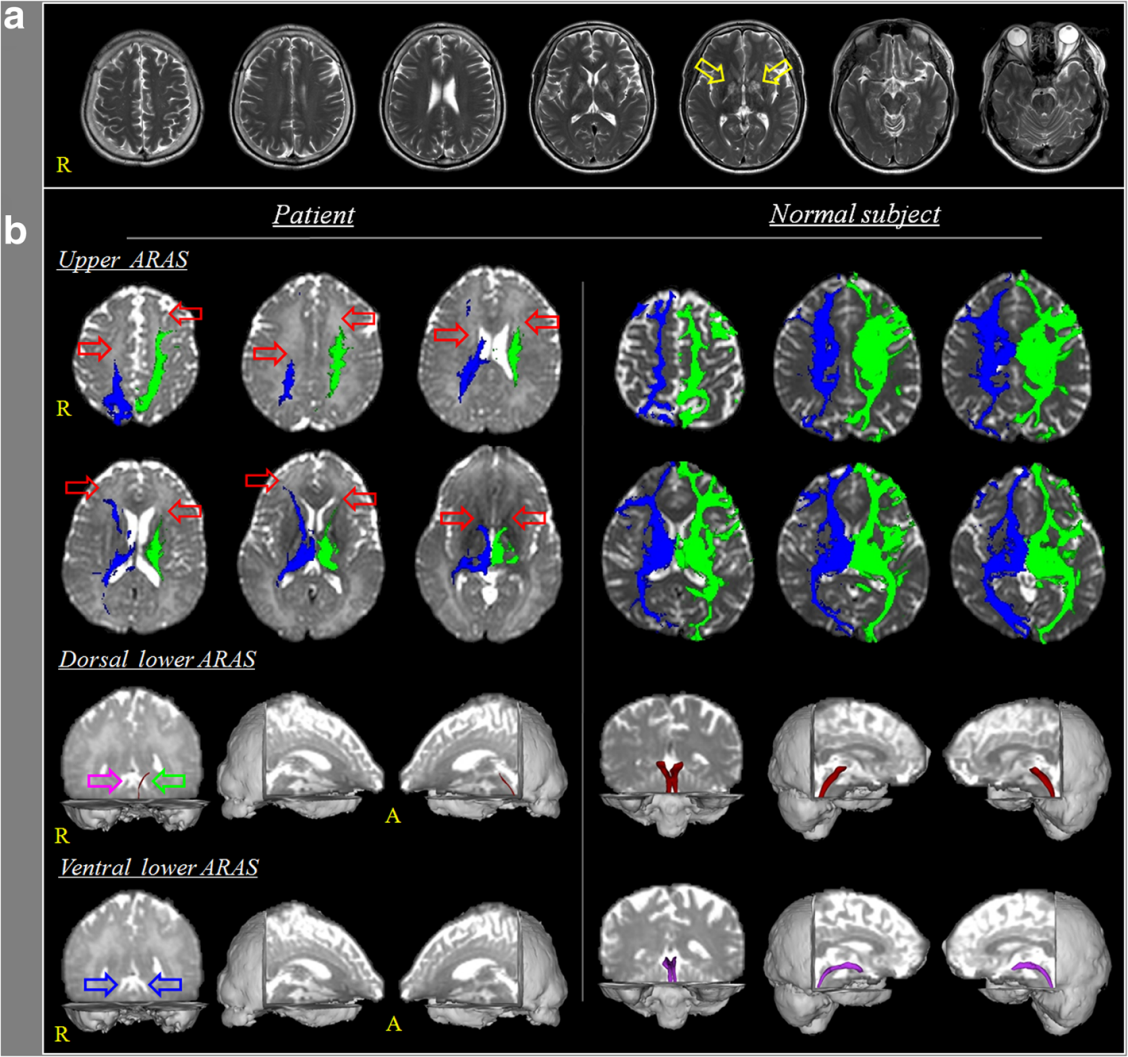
a Brain MR images at three weeks after onset show lesions in both basal ganglia (*yellow arrows*). b Results of diffusion tensor tractography (DTT) for the ascending reticular activation system (ARAS). On 6-week DTT, decreased neural connectivity of the upper ARAS between the intralaminar thalamic nucleus and the cerebral cortex is observed in both frontal cortices, basal forebrains, basal ganglia and thalami (*red arrows*). The dorsal lower ARAS between the pontine reticular formation and the intralaminar thalamic nucleus is not reconstructed on the right side (*purple arrow*) and thinning on the left side (*green arrow*). The ventral lower ARAS between the pontine reticular formation and the hypothalamus is not reconstructed on both sides (*blue arrows*). Results of DTT for the ARAS in a normal subject (53 year-old male). ARAS: ascending reticular activation system
